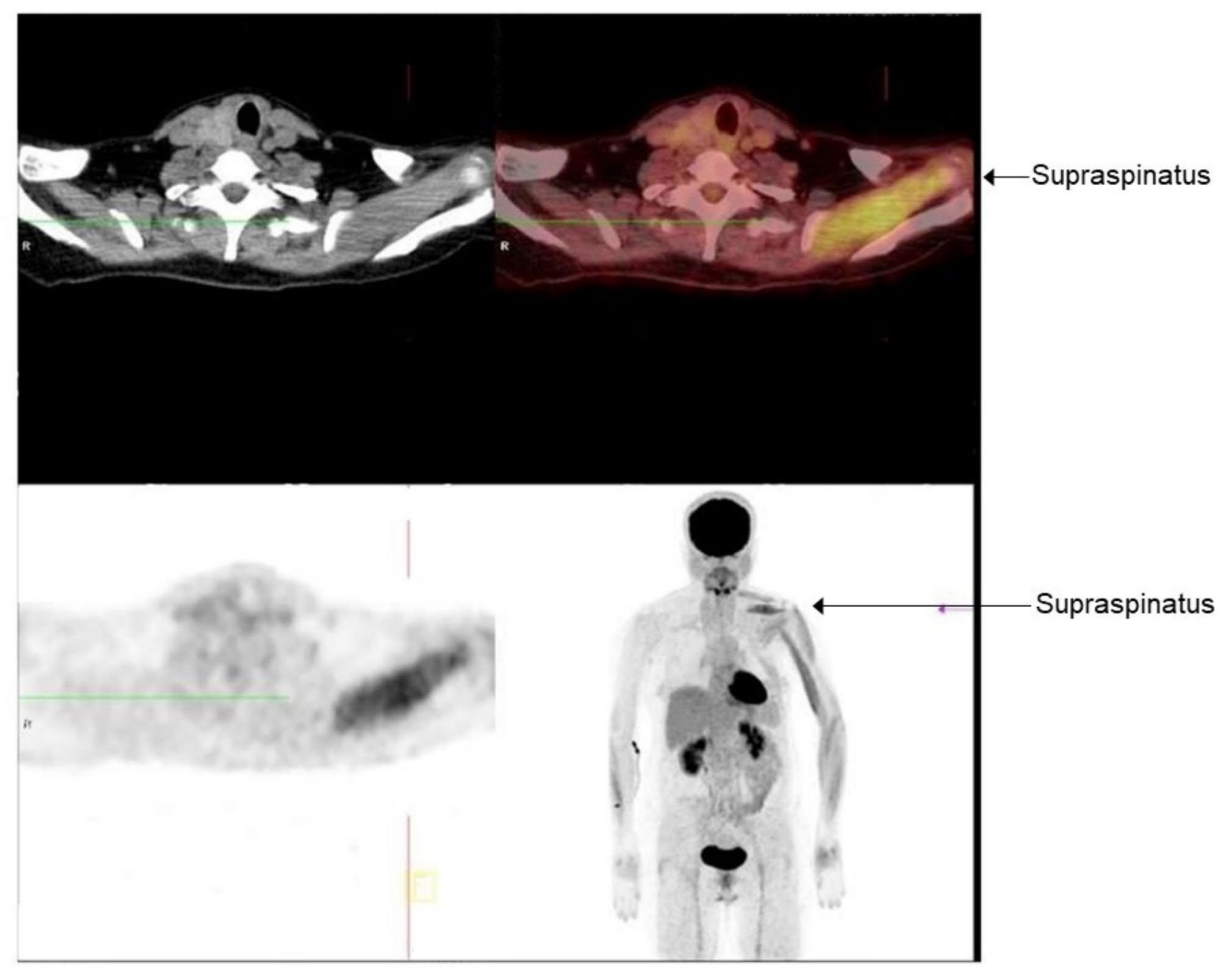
The positron emission tomography–computed tomography (PET-CT) scan of the patient. The image displays diffused areas of increased radioactivity uptake (score 1) in the supraspinatus, deltoid and bicep muscles of the left upper limb
